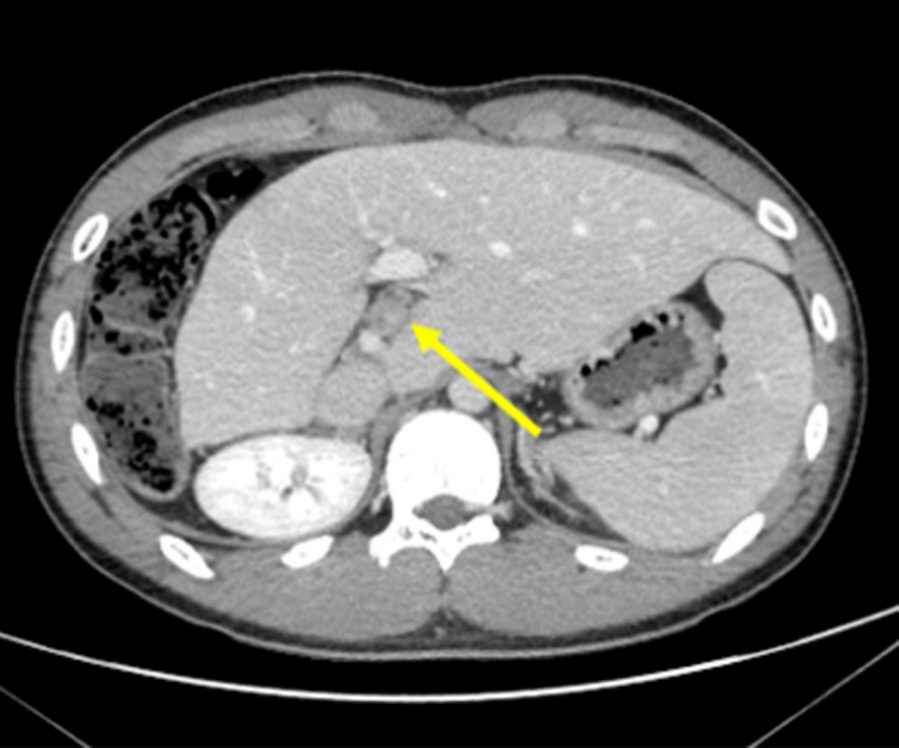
Abdominal CT shows a single lymphatic recurrence on the hepatoduodenal ligament (#12 lymph node) at 12 months after hepatectomy
